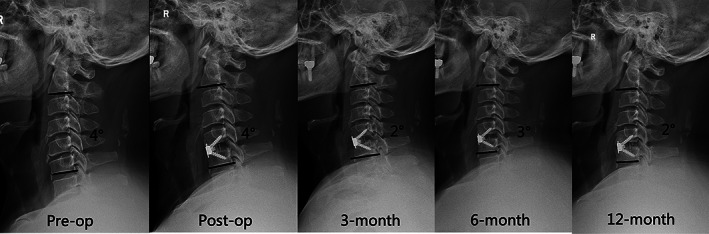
The follow‐up radiograph of patient employing traditional neck pillow (TNP). C7 slope (CL) was almost unchanged from pre‐operative 4° to post‐operative 4° and decreased to 2° at 12‐month follow‐up.
